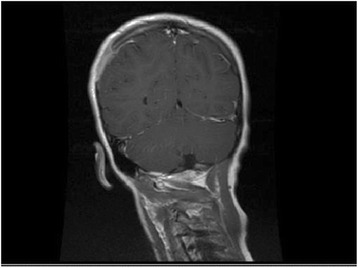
The cranial CT scan presented the solitary tissue mass overlying a skull lesion suggesting histiocytosis X in 12-years-old girl
